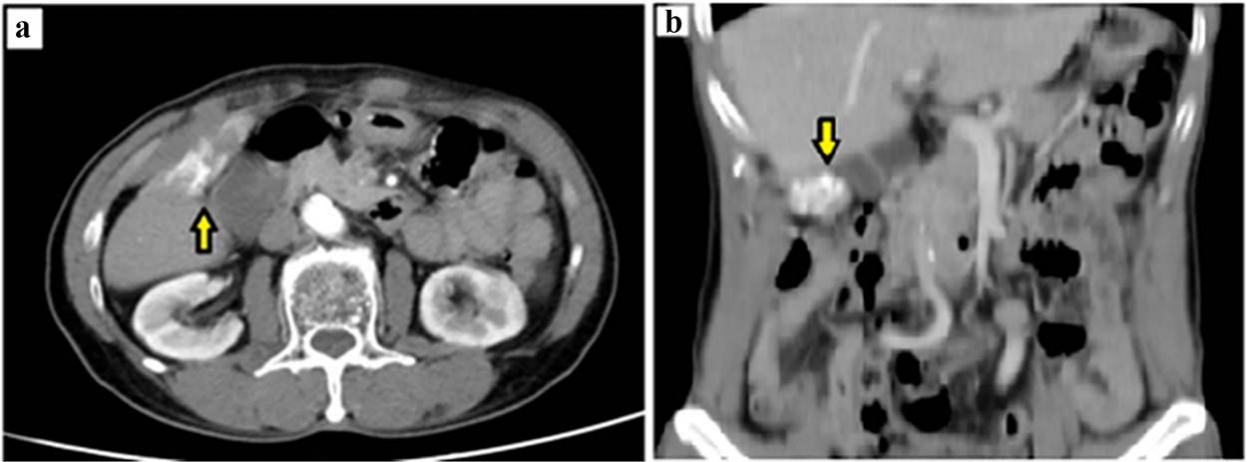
Preoperative contrast-enhanced CT demonstrating a calcified gallbladder fundus lesion suspicious for malignancy. (a) Axial CT shows a calcified mass at the gallbladder fundus with adjacent wall thickening (arrow). (b) Coronal CT reconstruction highlights the calcified lesion involving the gallbladder wall (arrow).
